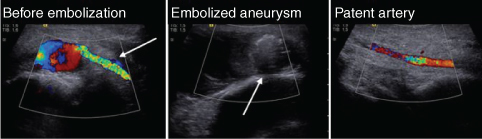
(a) Ultrasound Doppler image. Side branch of the anterior tibial artery (arrow) with connecting false aneurysm. (b) Ultrasound image of the aneurysm after thrombin injection showing no flow (arrow). (c) Ultrasound image showing normal flow in the anterior artery after thrombin injection.
